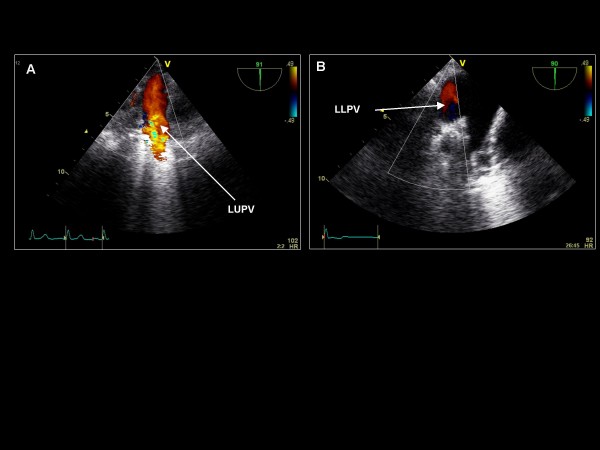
Imaging plane 90°, (A) Color Doppler demonstration of the left upper pulmonary vein (LUPV) and with further posterior probe rotation (B) the left lower pulmonary vein (LLPV).
